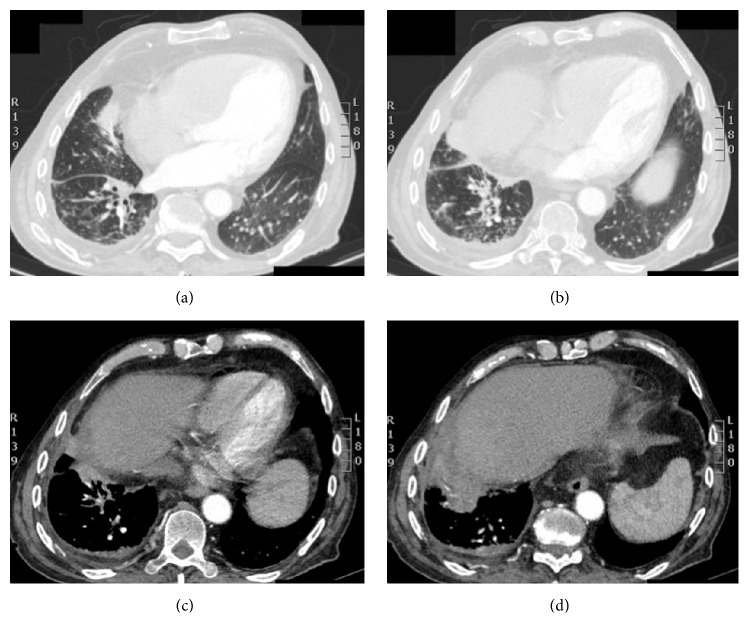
Control computed tomography of the chest. Almost complete resolution of the right lower lobe consolidation.
